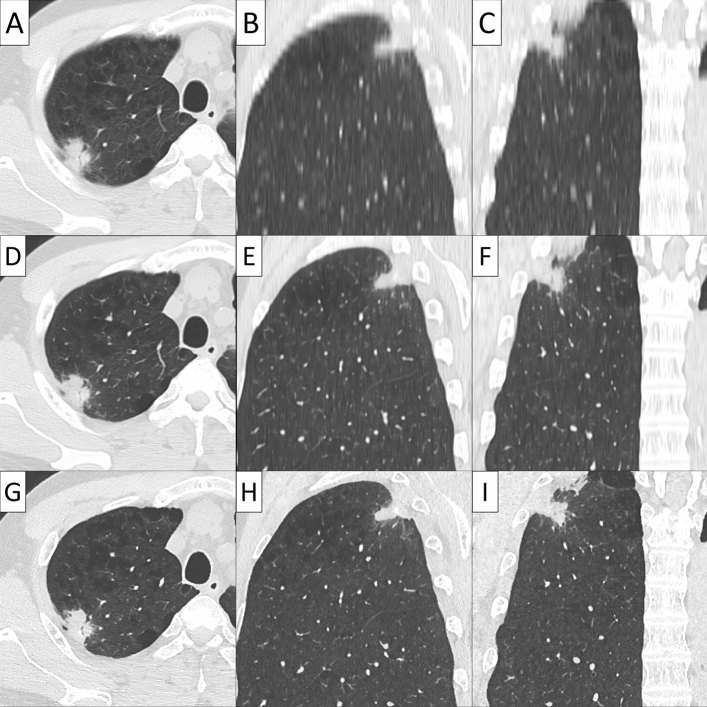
Solid adenocarcinoma in a 49-year-old male. (A) Axial view, (B) sagittal view, and (C) coronal view of conventional 5-mm thick CT. (D) Axial view, (E) sagittal view, and (F) coronal view of virtual thin-section CT (TSCT). (G) Axial view, (H) sagittal view, and (I) coronal view of real TSCT.
